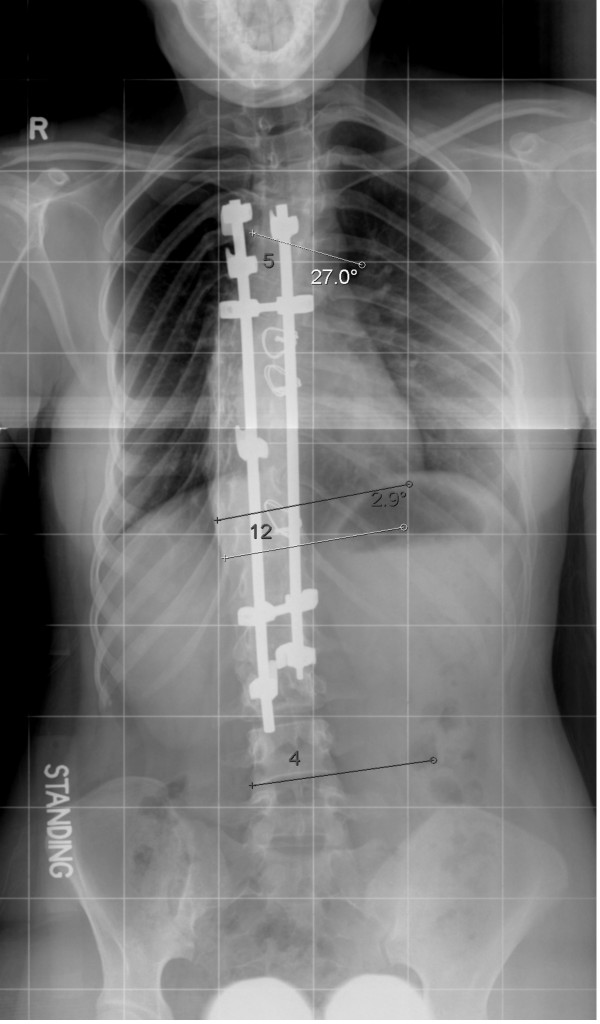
Standing radiograph 5 years after surgery.
